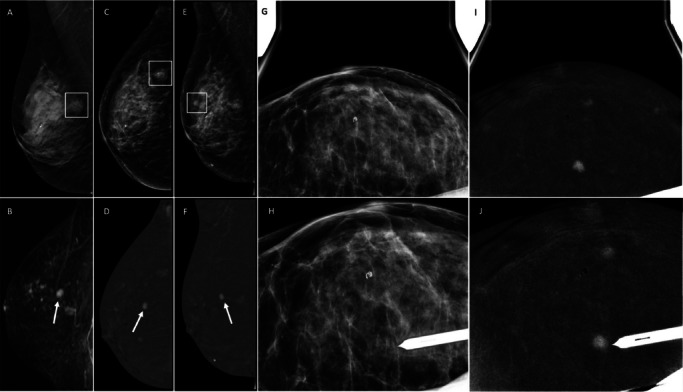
A 47-year-old patient with a history of right breast–conserving surgery 11 years ago and bilateral fibroadenomas (squares in A, C and E). Routine MRI follow-up (sagittal 3D Maximum Intensity Projection subtraction, B) showed a new right breast mass (arrow) not seen on second-look ultrasound or DBT (mediolateral oblique planes, A). Right breast recombined images (D and F) show the 8-mm enhancing mass (arrow), not seen on low-energy images (C and E). Right breast CEM-guided biopsy was performed (G–J). Pathology report: invasive lobular carcinoma. The patient underwent a mastectomy
